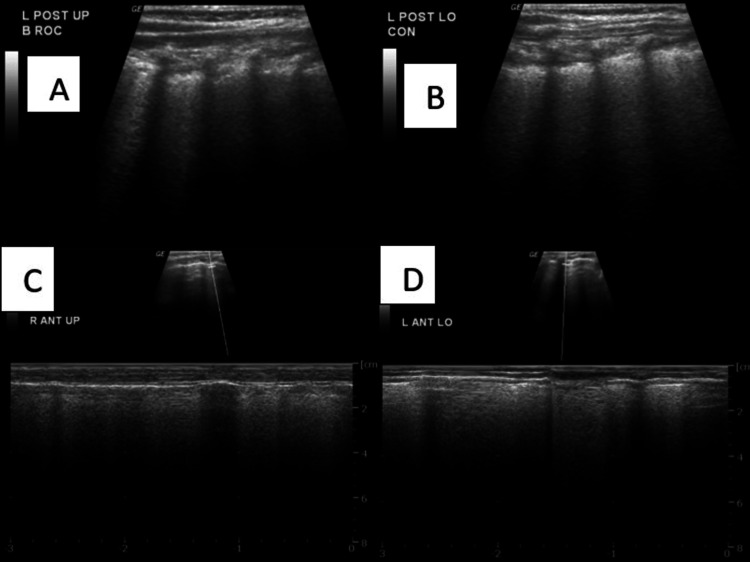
A) B-mode ultrasound in the left posterior upper region shows a thick irregular pleural line combined with a thick vertical hyperechoic stripe (C- line); B) B-mode ultrasound in the left posterior lower region shows a thick irregular pleural line combined with a thick vertical hyperechoic stripe (C- line); C,D) M-mode ultrasound confirming the presence of the C line. B mode: Brightness mode; M mode: Motion mode
